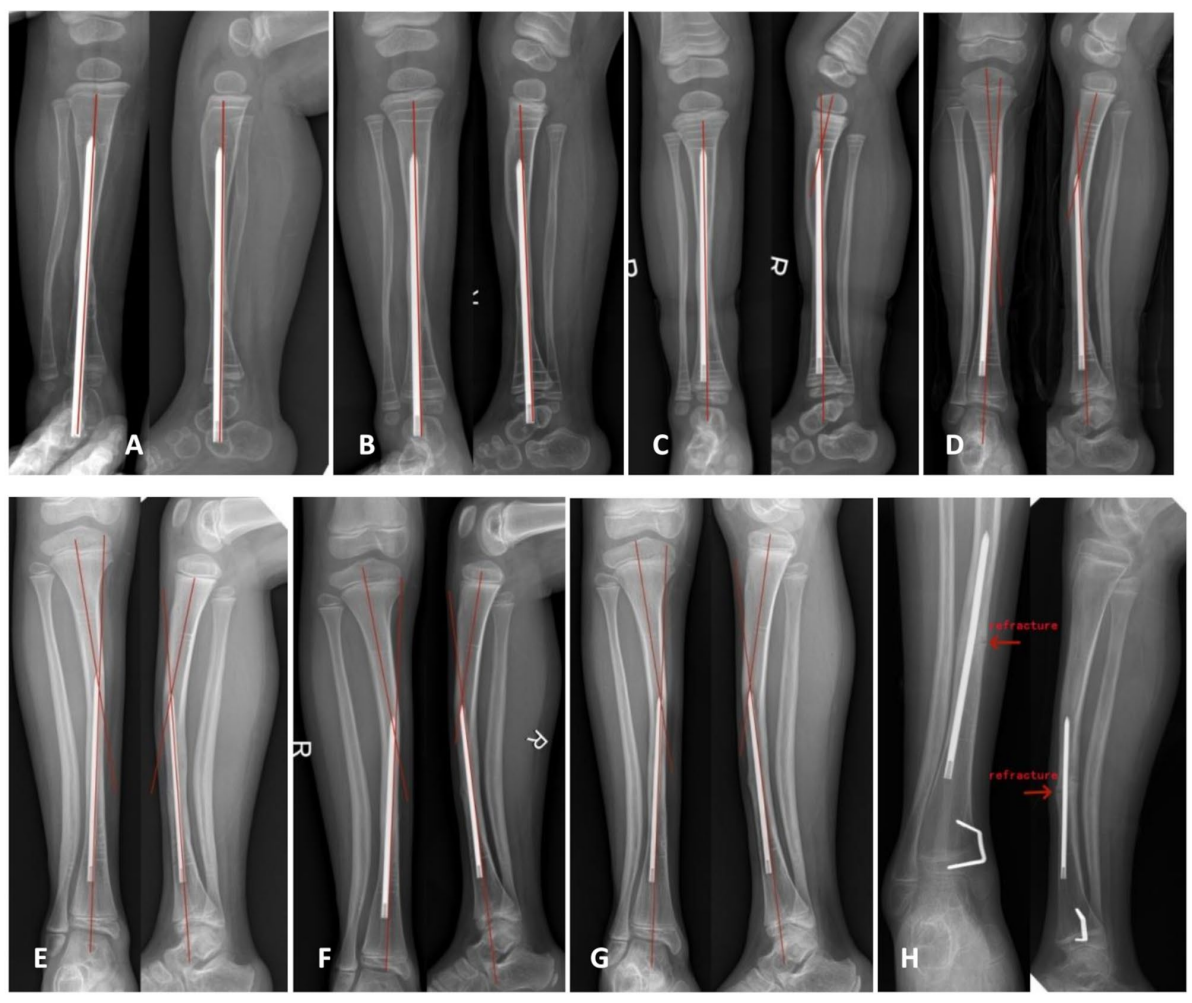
A case of tibial pseudarthrosis treated with combined surgery followed up for more than 10 years for X-ray changes after bone healing. It can be seen that the angular deformity of the tibial shaft gradually forms and worsens in the sagittal and coronal planes, accompanied by displacement of the tibial intramedullary rod and re fracture. A represented the anteroposterior and lateral views at six months after bone healing; B represented the anteroposterior and lateral views at 1 year after bone healing; C represented the anteroposterior and lateral views at one and a half years after bone healing, showing angular deformity of the tibial shaft in the sagittal plane; D represented the anteroposterior and lateral views at 4 years after bone healing, showing angular deformity of the tibial shaft in the sagittal and coronal planes; E represented the anteroposterior and lateral views at 6 years after bone healing, showing angular deformity of the tibial shaft in the sagittal and coronal planes; F represented the anteroposterior and lateral views at 7 years after bone healing; G represented the anteroposterior and lateral views at 8 years after bone healing; H represented the anteroposterior and lateral views at 10 and a half years after bone healing, showing angular deformities of the tibial shaft in the sagittal and coronal planes, accompanied by displacement of the tibial IM rod and re fracture
